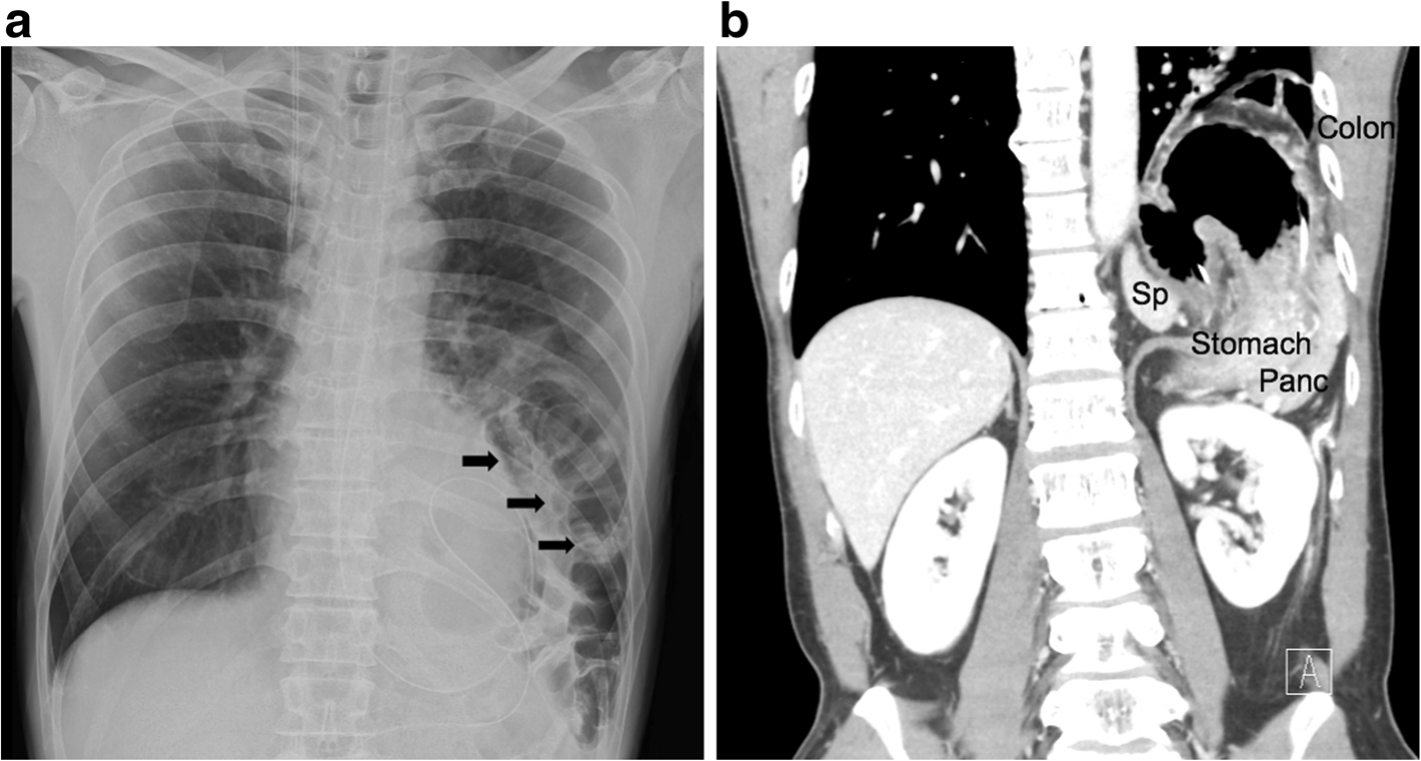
Chest X-ray showing colon gas and a nasogastric tube above the left diaphragm. Arrows showed the herniated colon (a). Coronal CT imaging revealed herniation of the stomach, pancreas, spleen, and colon (b)
